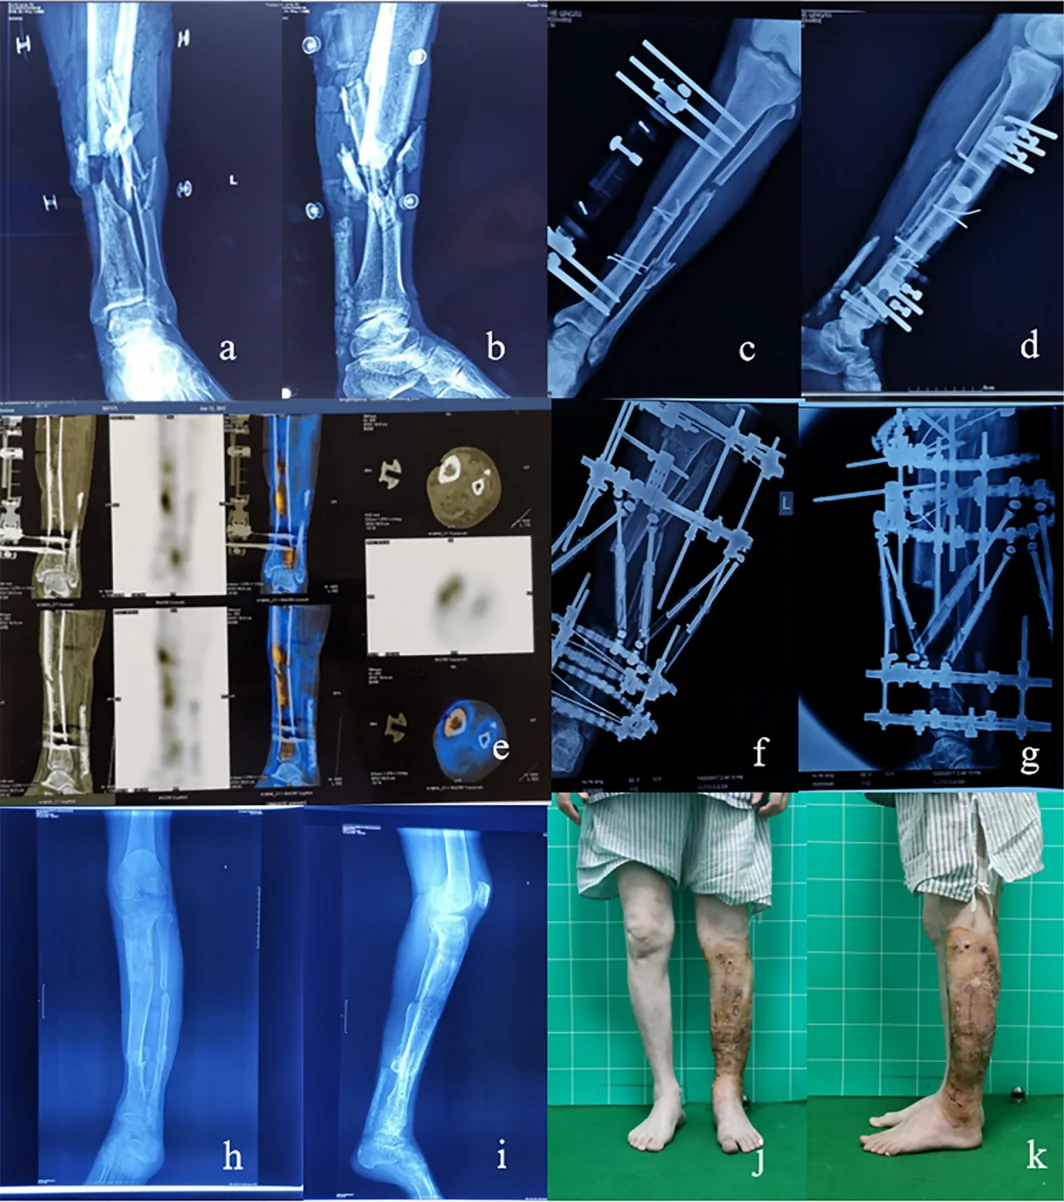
A 39‐year‐old male with ITBD‐STD was managed via the BT technique. (a, b) X‐ray images of the injured tibia at the time of injury. (c, d) Postoperative x‐ray showing unilateral external fixator fixation of the left tibia performed at an outside hospital emergency department. (e) SPECT/CT of the left lower leg showing increased bone metabolism in the middle and lower segments of the tibia, suggestive of an infectious lesion. (f, g) Postoperative x‐ray showing resection of the infected middle and lower tibial segment, fixation with a circular external fixator, and proximal tibial osteotomy. (h, i) Postoperative x‐ray showing external fixator removal, adequate mineralization at the bone lengthening site, and solid union at the docking site. (j, k) Clinical follow‐up photographs taken 1 month after removal of the circular external fixator.
